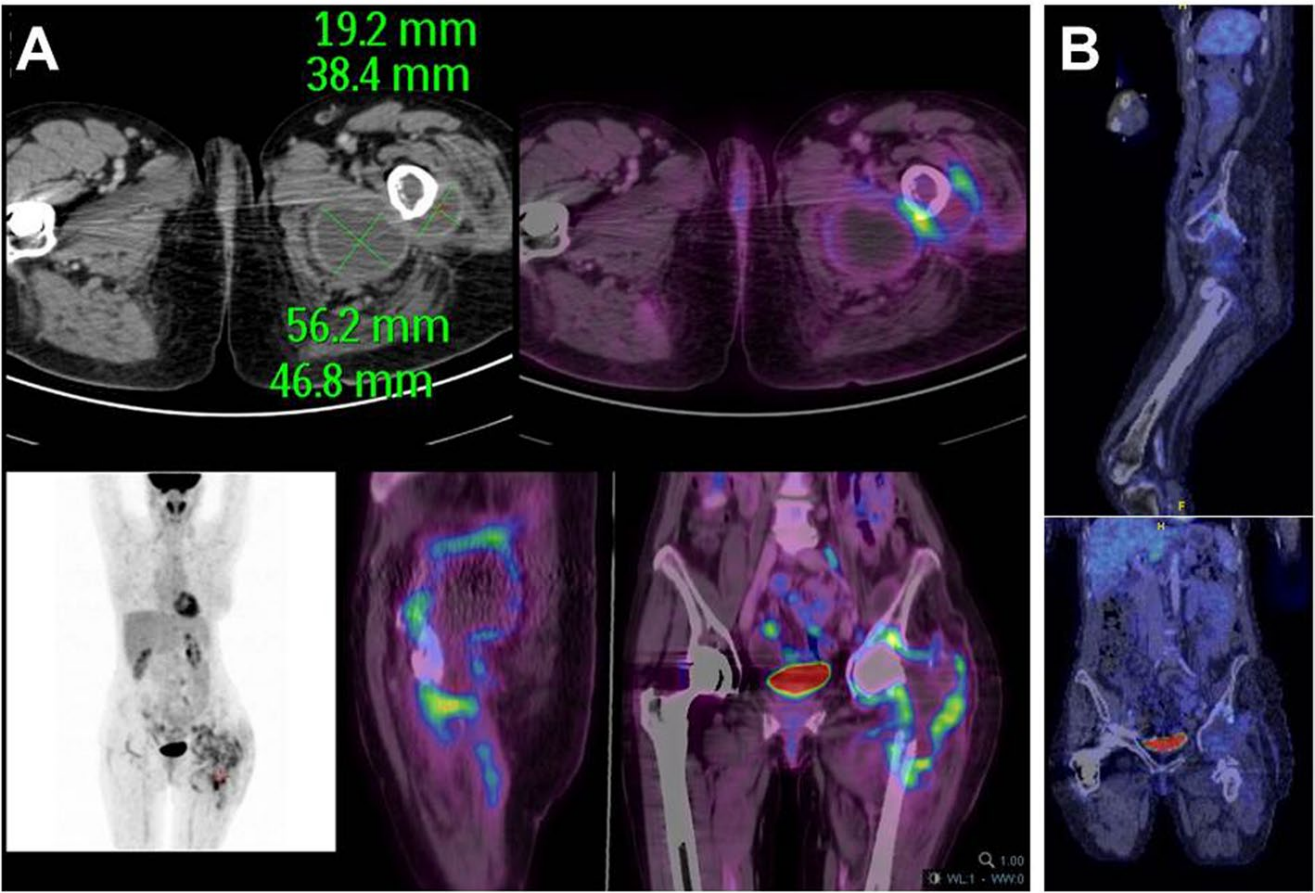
Positron emission tomography/computed tomography (PET-CT) performed ~ 3 months after the prosthesis explantation, showing a large ilio-psoas muscle collection (47 × 56 mm) communicating with numerous periprosthetic collections of the left hip (panel A). A second PET-CT was performed in June 2014, after the third surgical procedures and at ~ 6 month of rifampin and ofloxacin combination therapy, showing no persistent inflammatory process of the left hip and thigh (panel B)
